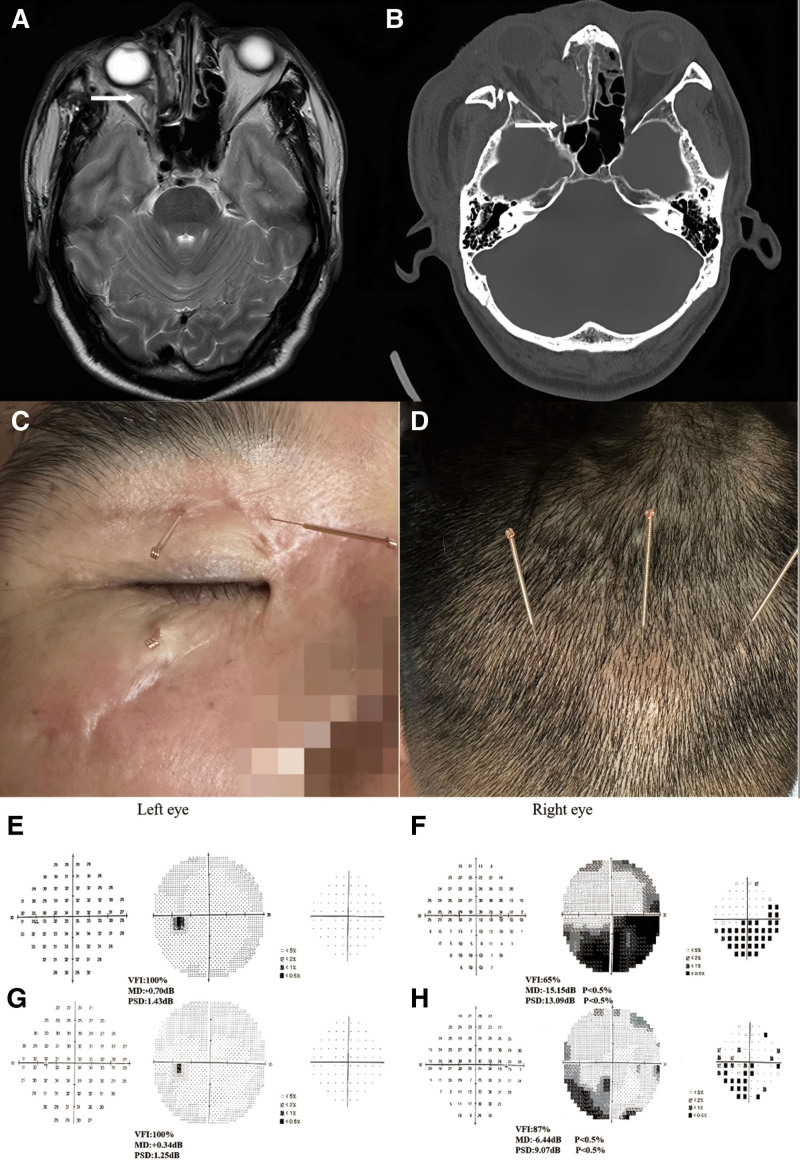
MR and CT findings showing a fracture of the optic canal, injure and swelling of the optic nerve on the right side (A and B). Humphrey visual field examination results in the patient (C and D). First examination of the patient’s binoculus (E and F); second examination of the patient’s binoculus (G and H). CT = computed tomography.
